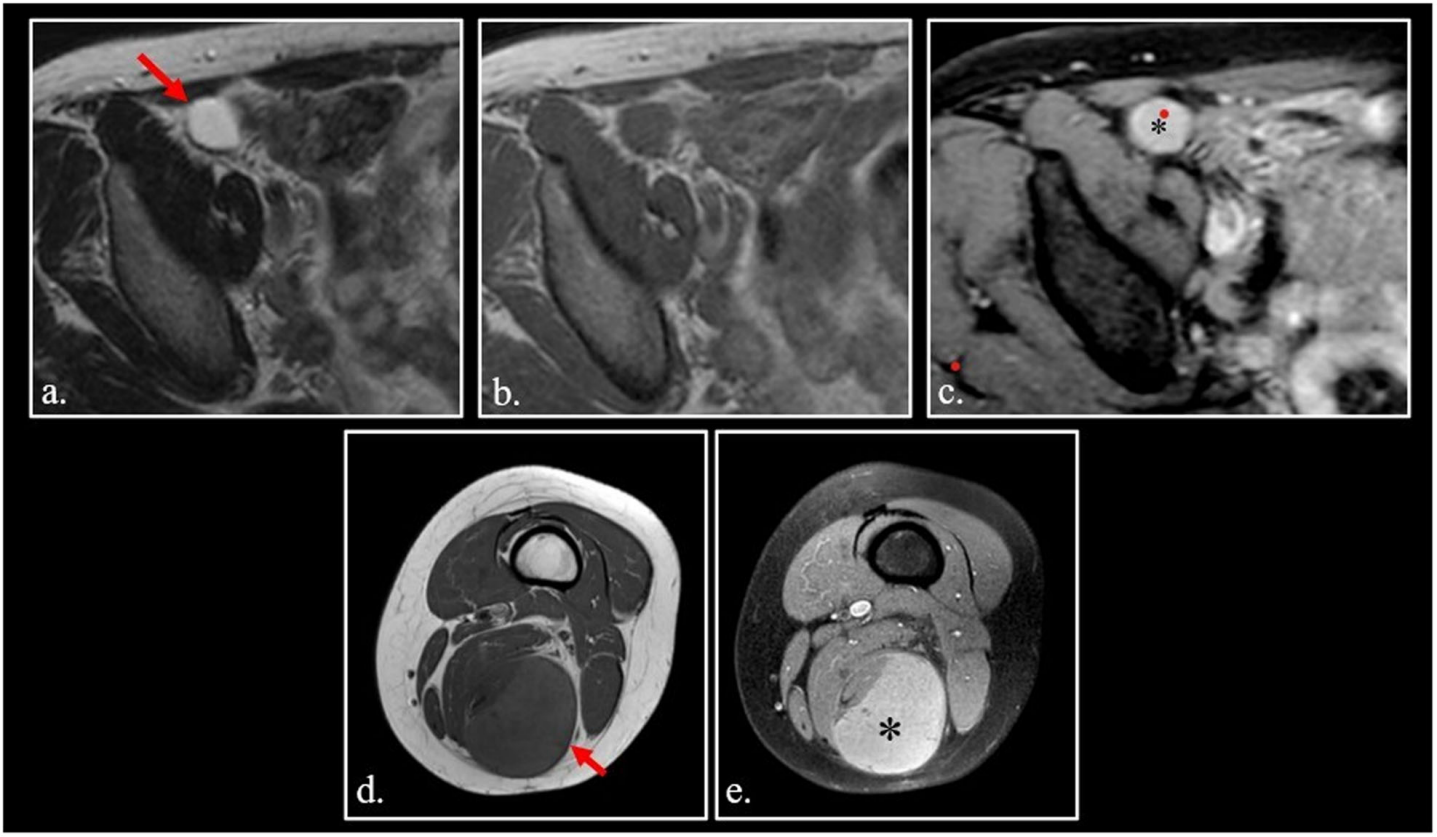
a–c Appendicular mucocele showed on FSE T2-weighted (a), T1-weighted (b) and T1-weighted fat-sat (c) scans. Note the rescaling phenomenon on T1-weighted fat-sat scan (asterisk). d, e Unenhanced FSE T1-weighted (d) and T1-weighted fat-sat (e) of a 35-year-old female with rapidly growing clear cell sarcoma of the left thigh (arrow). Note the rescaling phenomenon in (e) (asterisk)
